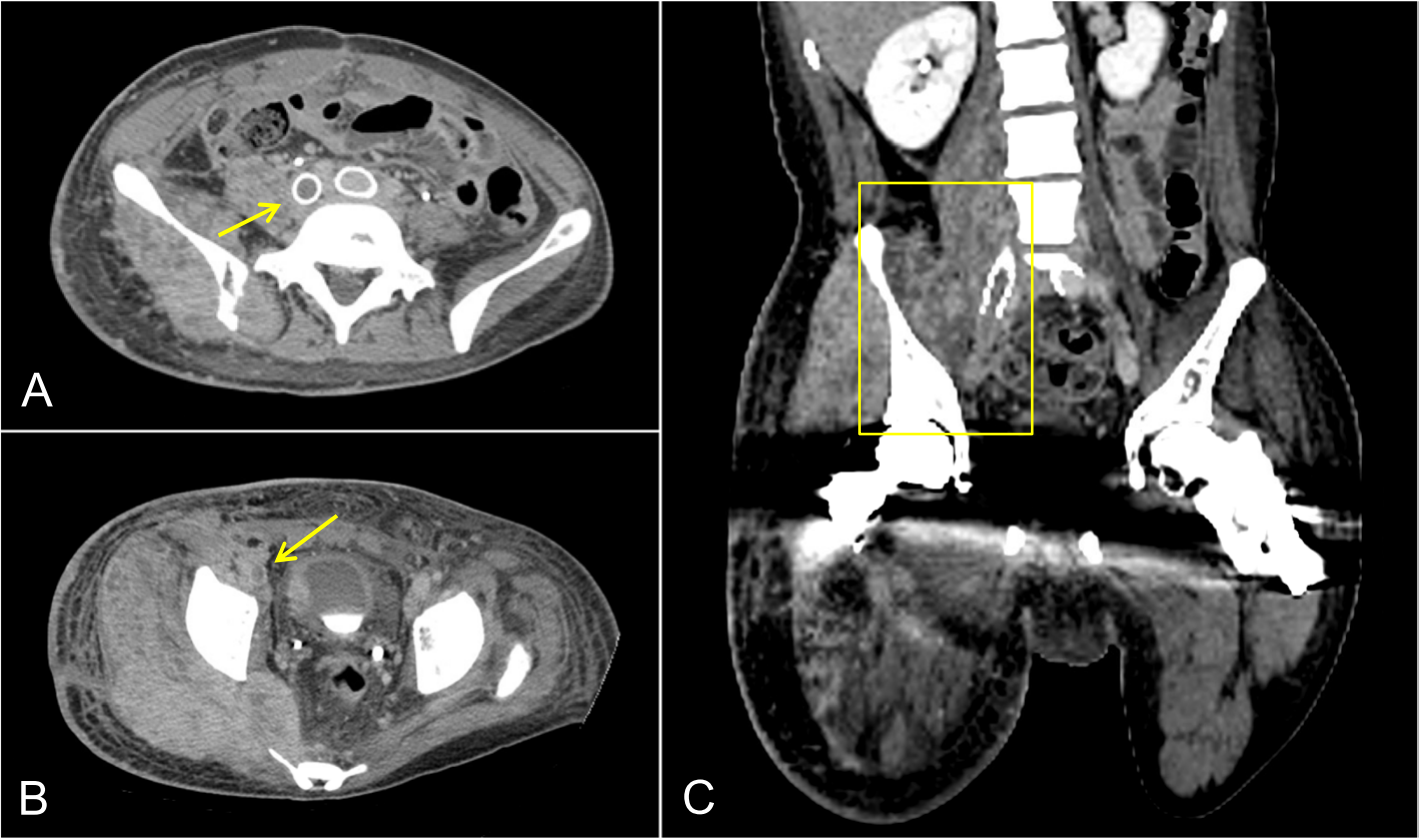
A and B Axial CT venogram demonstrates recurrent thrombosis of the right common iliac vein stent with extension of thrombus to the right lower extremity (arrows). C Coronal reformat of the CT venogram depicts tumor extension and mass effect on the right pelvic veins (box)
